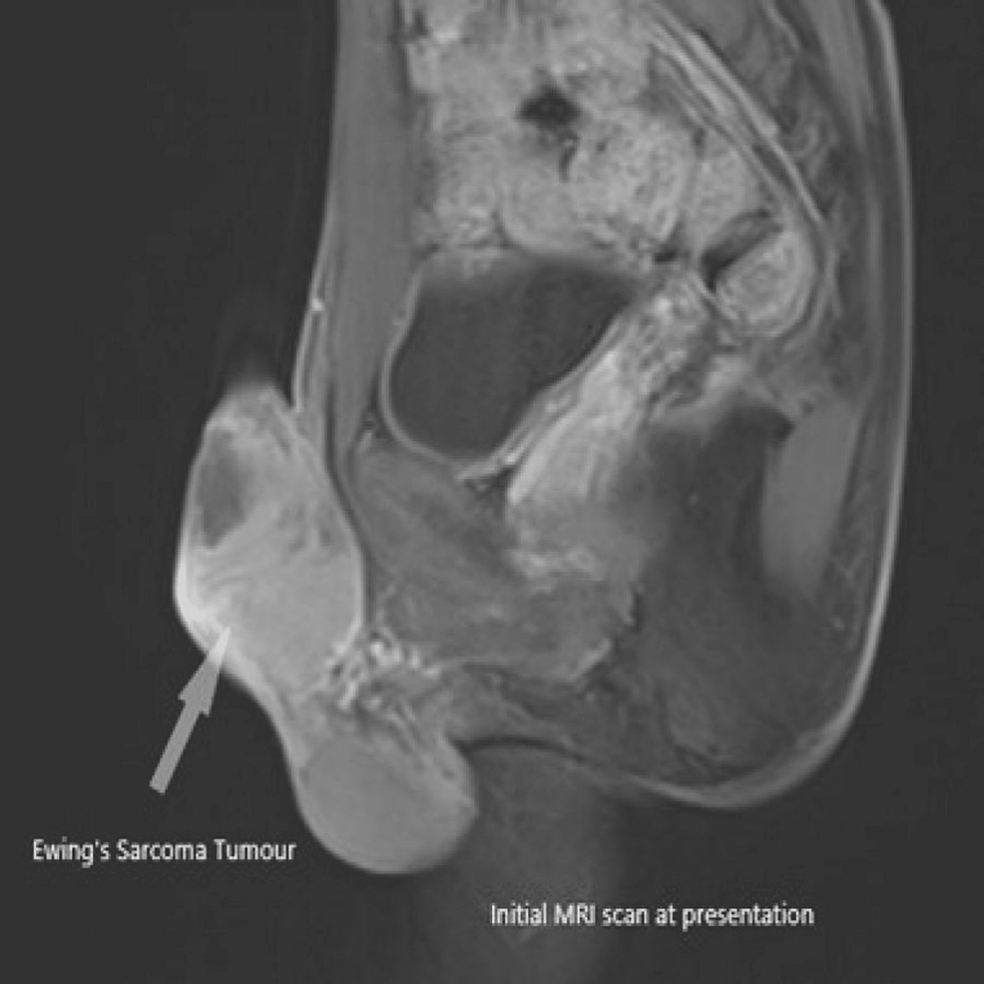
Initial MRI scan at presentation (sagittal plane).
